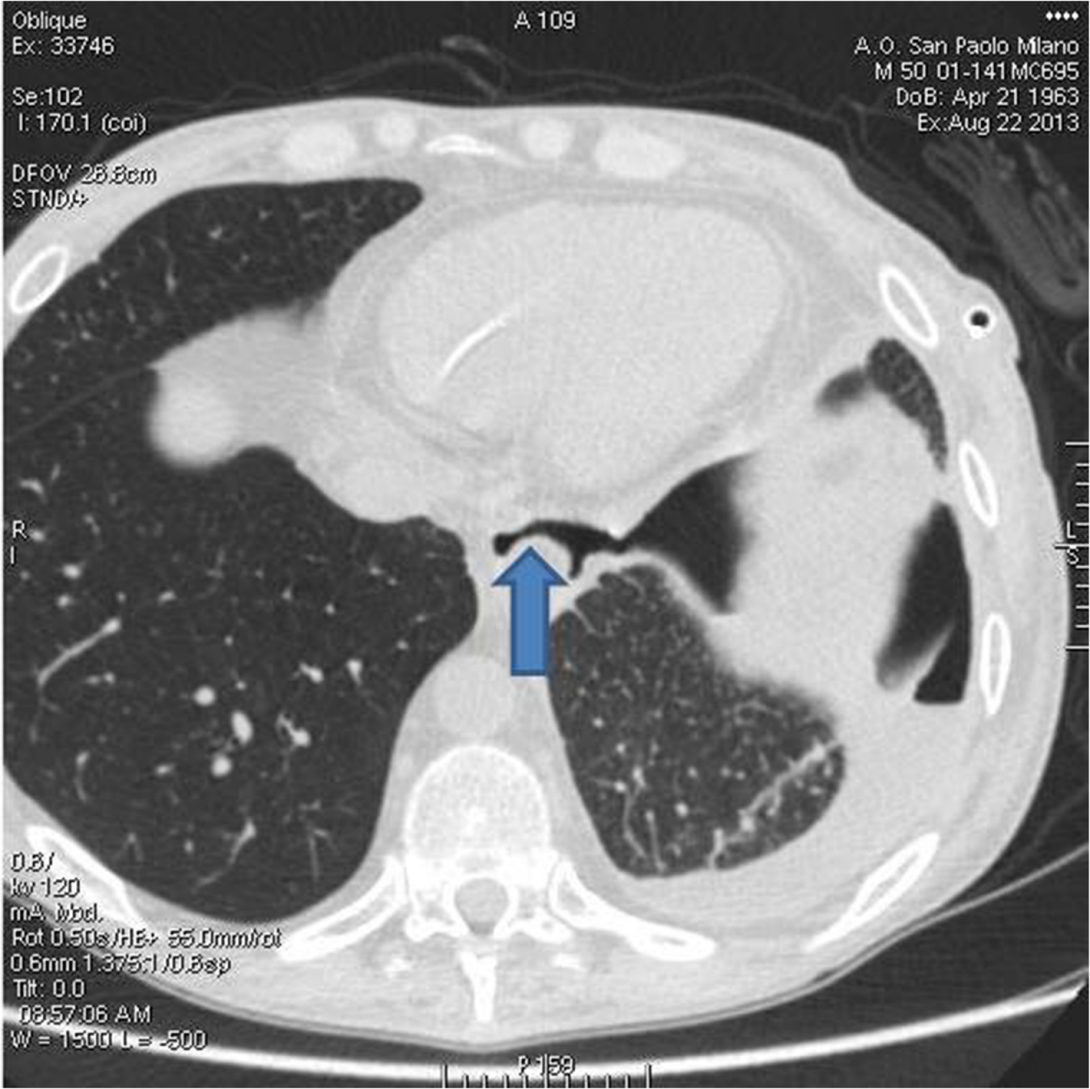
Contrast-enhanced CT-scan showing an air-filled communication between trachea and mediastinum
